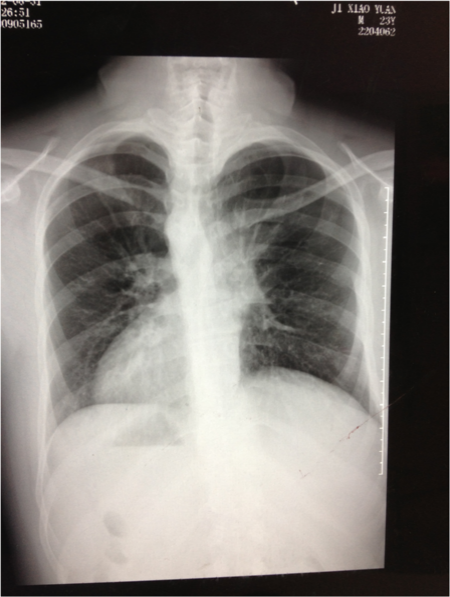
Chest X-rays showing recovery after six months follow-up
